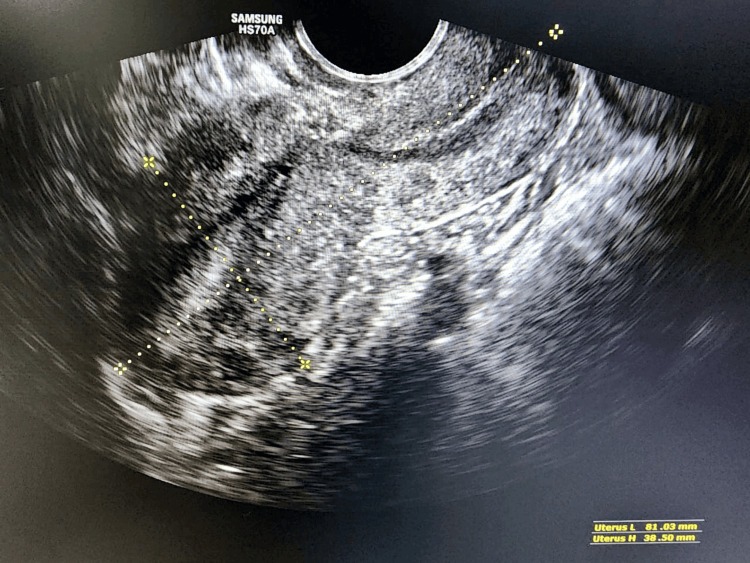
Showing ultrasonography post operative Isthmocele
